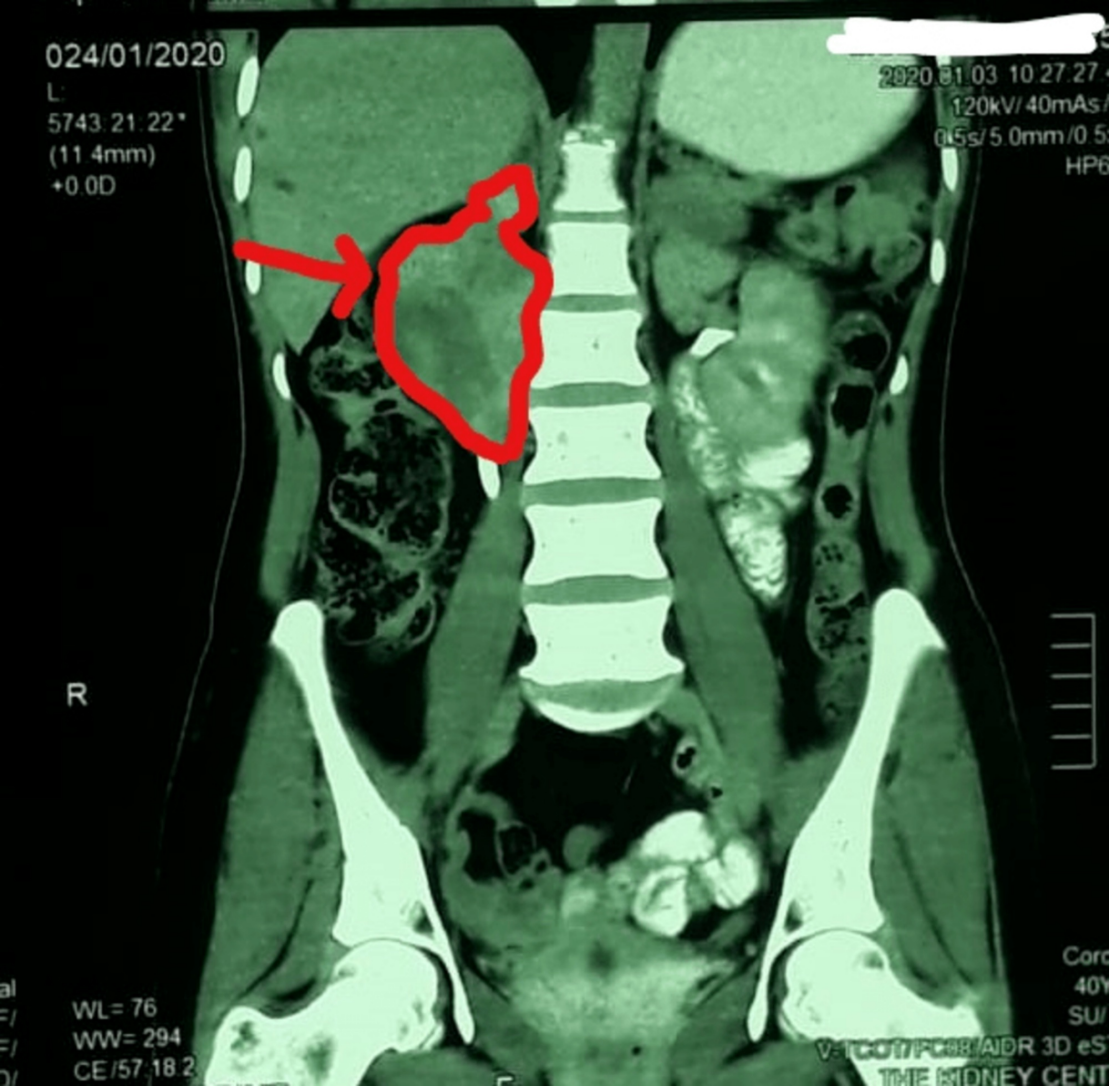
CT scan (coronal view) of the abdomen showing a right-sided mass.
